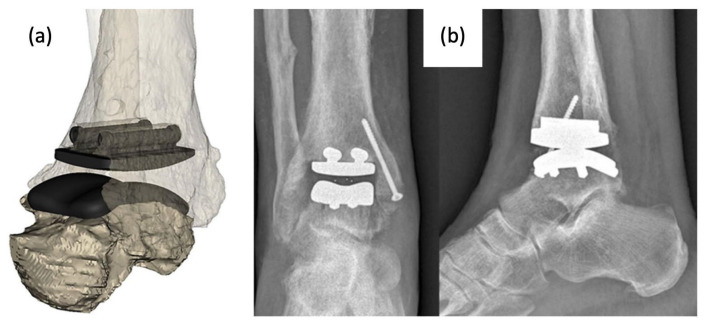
(a) Computer model of custom-designed implant on patient CT scan rendered bone model and (b) anterior–posterior and lateral X-rays of implanted AM TAA implant [36].
